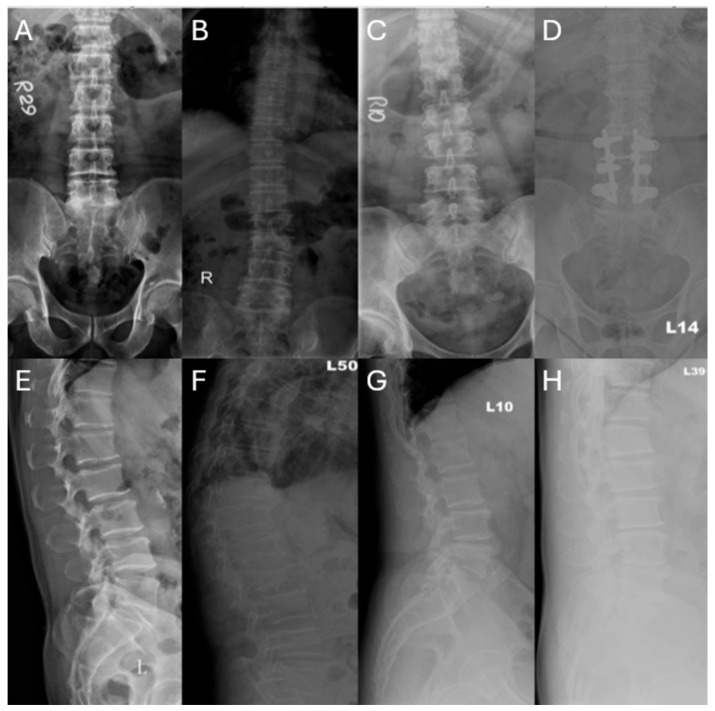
Definition of image quality on AP and Lat plain radiographs. (A) Standard AP radiograph meeting all quality criteria. (B) AP radiograph with incomplete coverage of the pelvic brim. (C) AP radiograph with off-center positioning. (D) AP radiograph with overexposure. (E) Standard Lat radiograph of the lumbosacral spine meeting all quality criteria. (F) Lat radiograph with incomplete sacral coverage. (G) Lat radiograph with incomplete T11 coverage. (H) Lat radiograph with overexposure.
